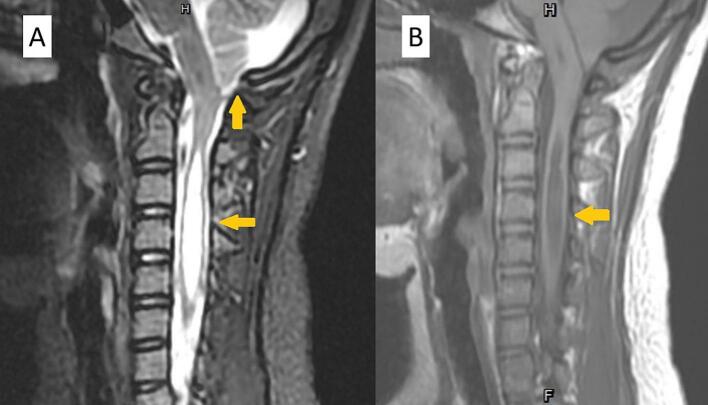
A. A sagittal T2-weighted magnetic resonance imaging (MRI) scan of the cervicothoracic region reveals a hyperintense signal within the spinal cord at the C2 to C6 levels, accompanied by observable spinal cord enlargement. B. A sagittal T1-weighted MRI of the cervicothoracic region demonstrates a hypointense signal at the C2 to C6 spinal cord levels, as well as spinal cord enlargement. Additionally, herniation of the cerebellar tonsils through the foramen magnum is observed.
